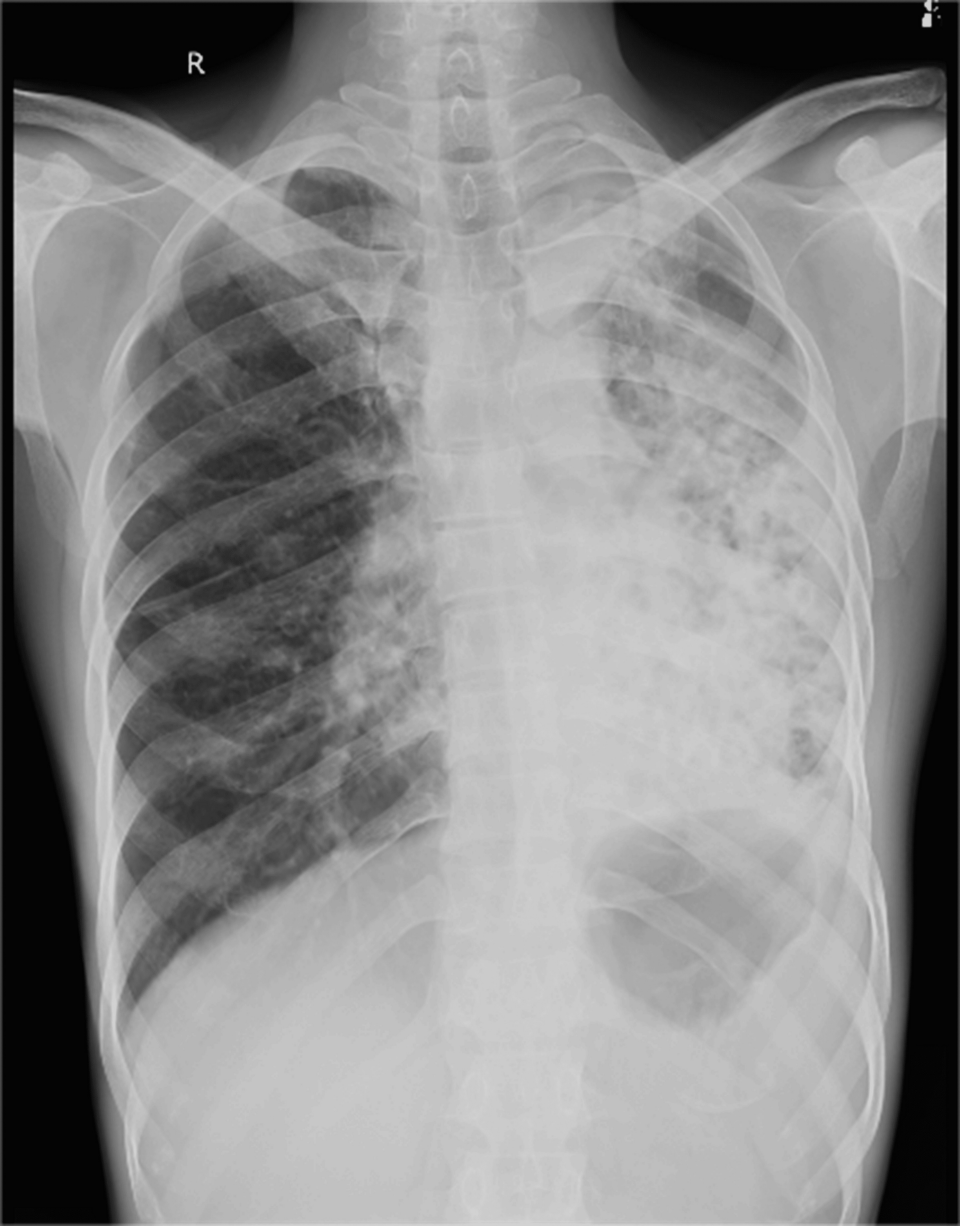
Chest X-ray suggestive of fibrocavitary lesions on the left side
